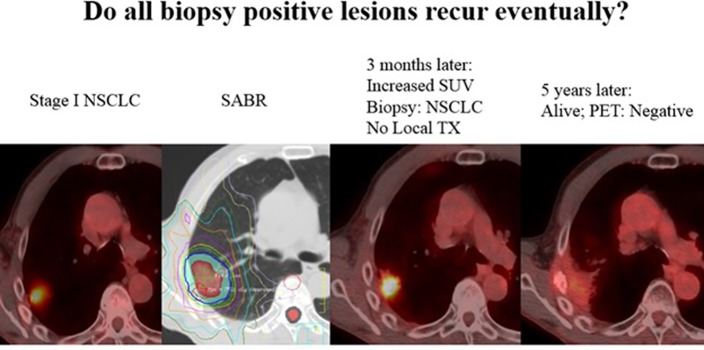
Not all “positive” biopsies months after SABR lead to recurrence. Here, a 71 year old male with stage I NSCLC was treated with SABR, 50Gy in 4 fractions. A routine surveillance PET/CT three months later after SABR showed increased SUV uptake. A biopsy was performed and was positive for “residual cancer.” The patient was sent for evaluation of surgery and thermal ablation but neither were recommended due to poor performance status. The patient was followed, and 5 years later his tumor has disappeared and he has been without disease or any signs of local recurrence. PET uptake has also resolved without any treatment. Although a biopsy and PET may suggest viable disease, tumor cells can often be dead/dying after SABR and lead to no recurrence.
